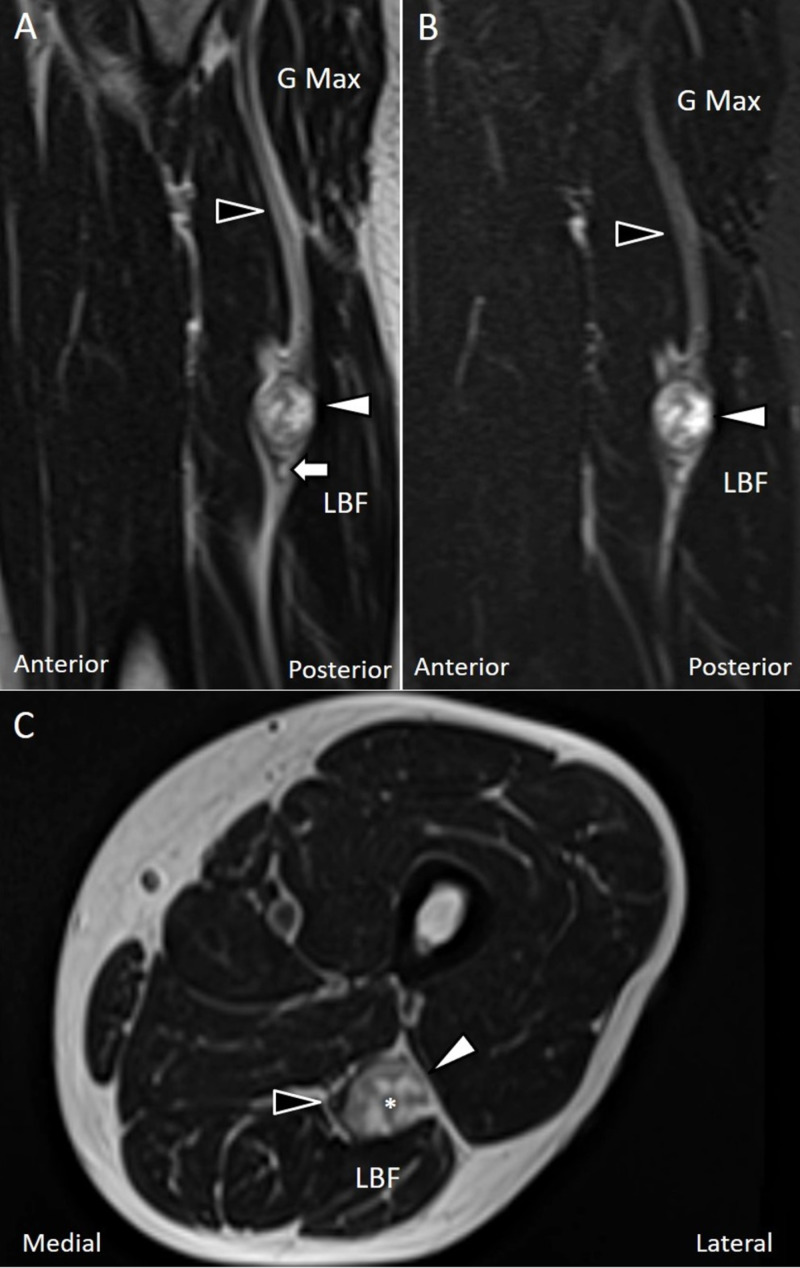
MRI of the sciatic schwannoma A well-defined tumor of dimensions 2.3 × 1.8 × 2.3 cm was noted in the posterior compartment of the left thigh under the T2-weighted non-contrast (A) and T1-weighted contrast-enhanced MRI (B). On the axial plane, the tumor was located beside the left sciatic nerve (C). Black arrowheads: sciatic nerve; white arrowheads: schwannoma; white arrow: split-fat sign; asterisk: fascicular sign. G Max: gluteus maximum; LBF: long head of biceps femoris.
